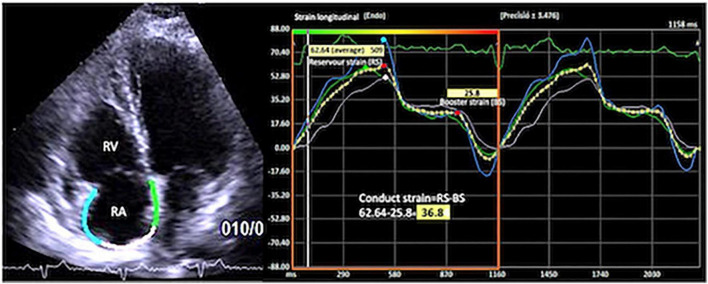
Apical four-chamber view showing the right atrial global longitudinal strain (RA-GS) with the measurement of reservoir, conduit, and contractile strain phases.
